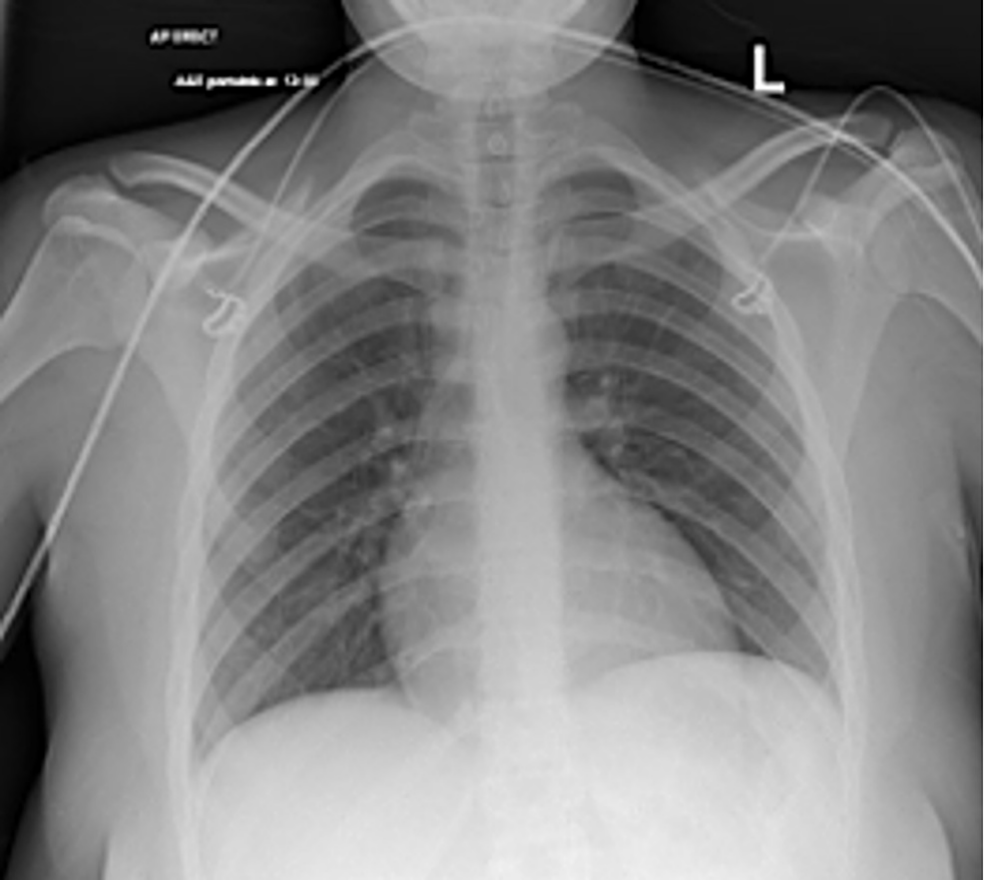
On admission, plain film anteroposterior erect chest radiograph.
